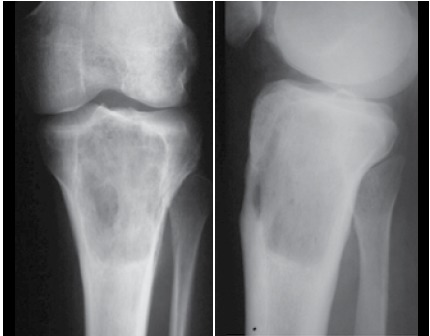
X-ray image of a primary bone lymphoma in the proximal tibia (prior to chemotherapy).
